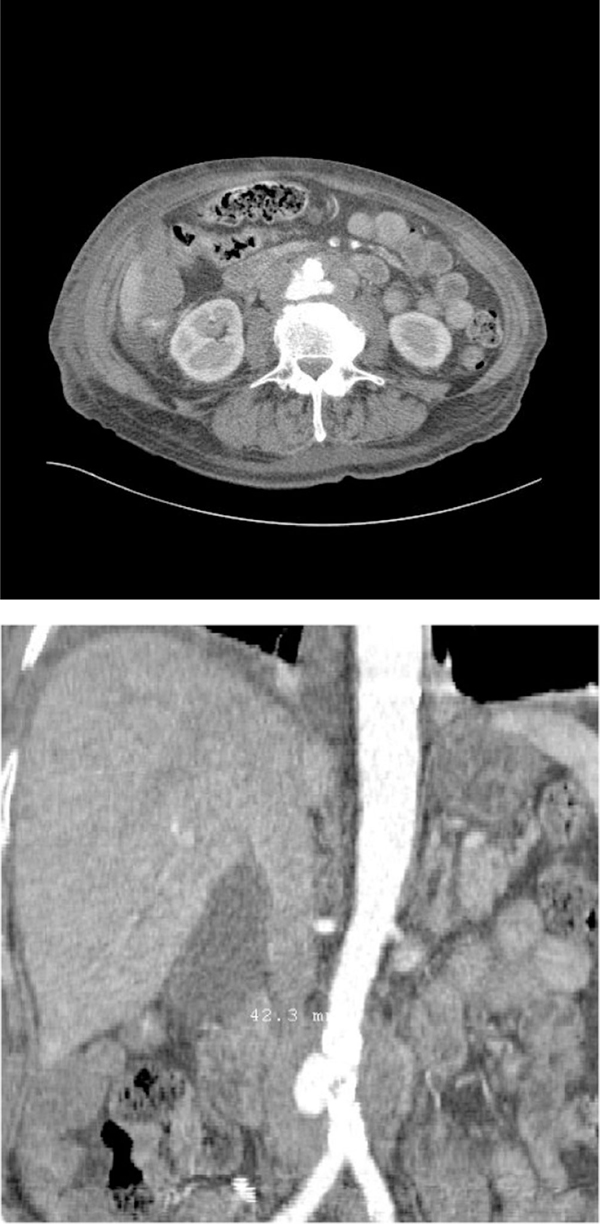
(A) A computed tomography scan showing localised perforation of infrarenal aorta and stranding of surrounding tissue. (B) Two-dimensional coronal reconstruction of lower abdomen demonstrating position and size of leak.
